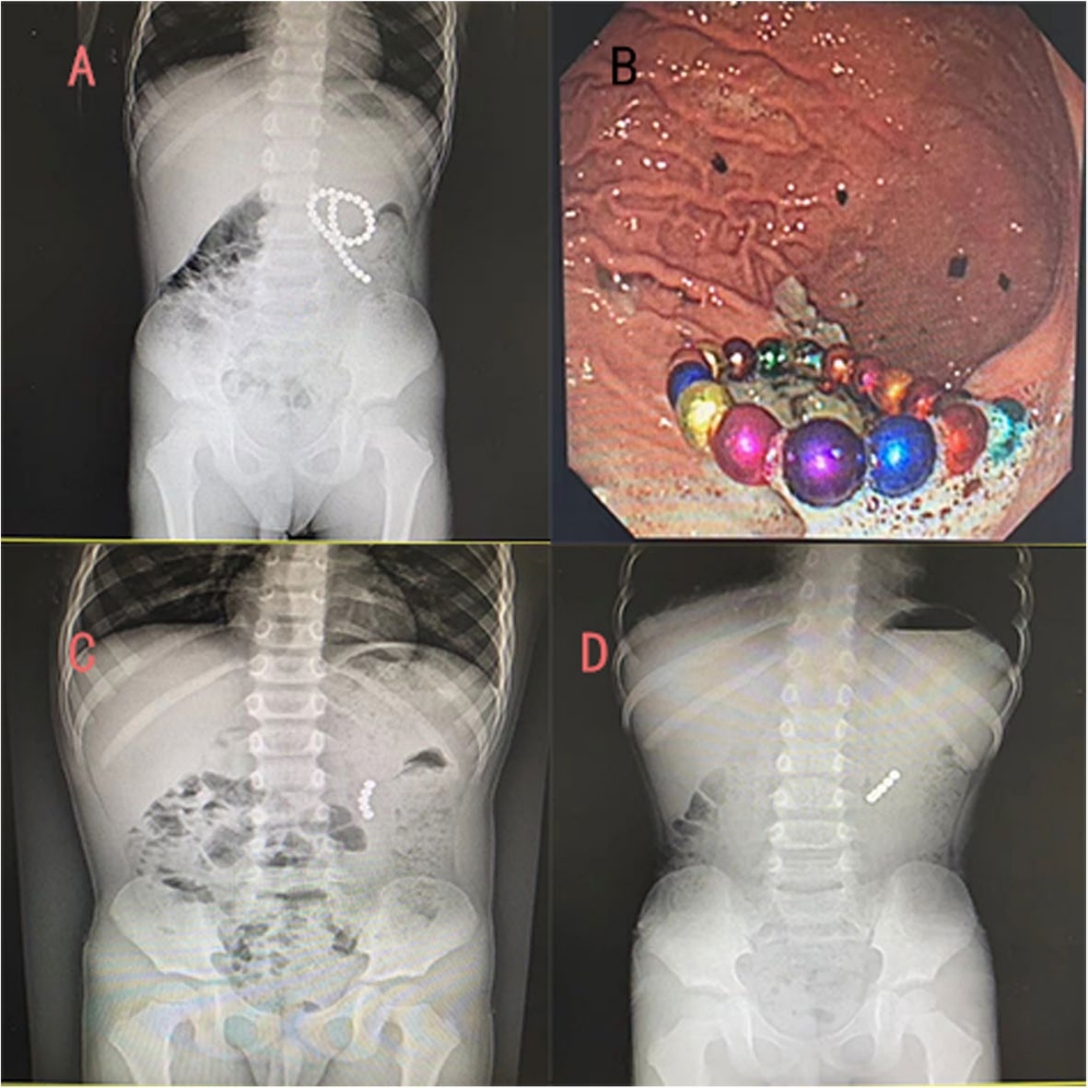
The x-ray showed that the foreign bodies were in the left upper abdomen, so gastroscope was performed. It was found that there were 26 foreign bodies in the stomach. After removal under gastroscope, the patient had no obvious abdominal symptoms, but continuous x-ray examination showed that the position of foreign bodies had no significant change. Considering the difficulty of self-discharge, the foreign body was surgically removed
